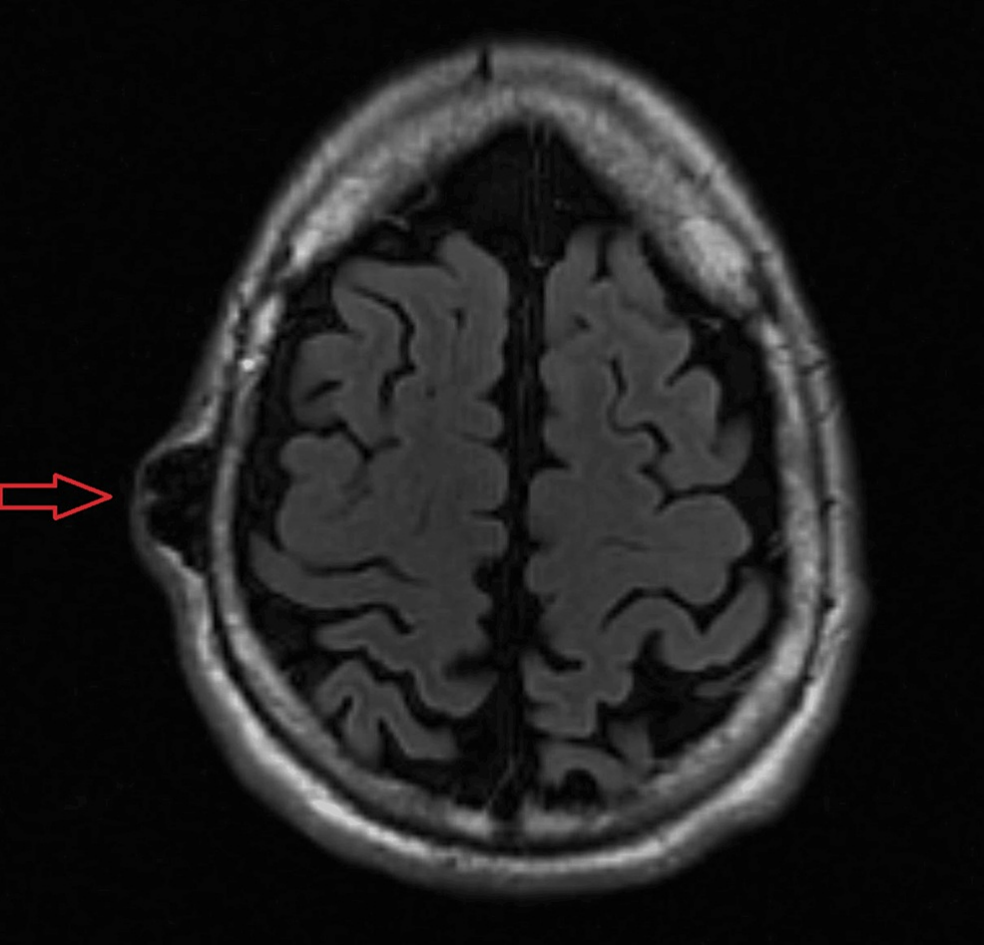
Axial T2 weighted magnetic resonance imaging (MRI) image shows a tangle of flow voids in the right parietal scalp (arrow) indicating high-flow vessels.
